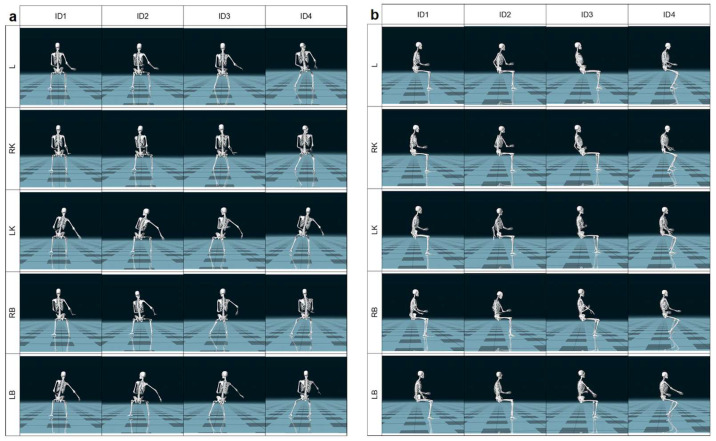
Identification of 5 critical physicians’ body positions in coronal (a) and sagittal plane (b). ID1–ID4—sonographer identification; L—liver; LB—left breast; LK—left kidney; RB—right breast; RK—right kidney.
